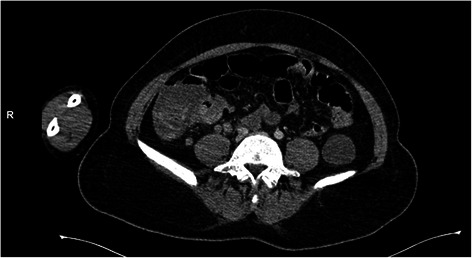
Abdominal computed tomography of the abdomen showing an infiltrative aspect and regional nodes
